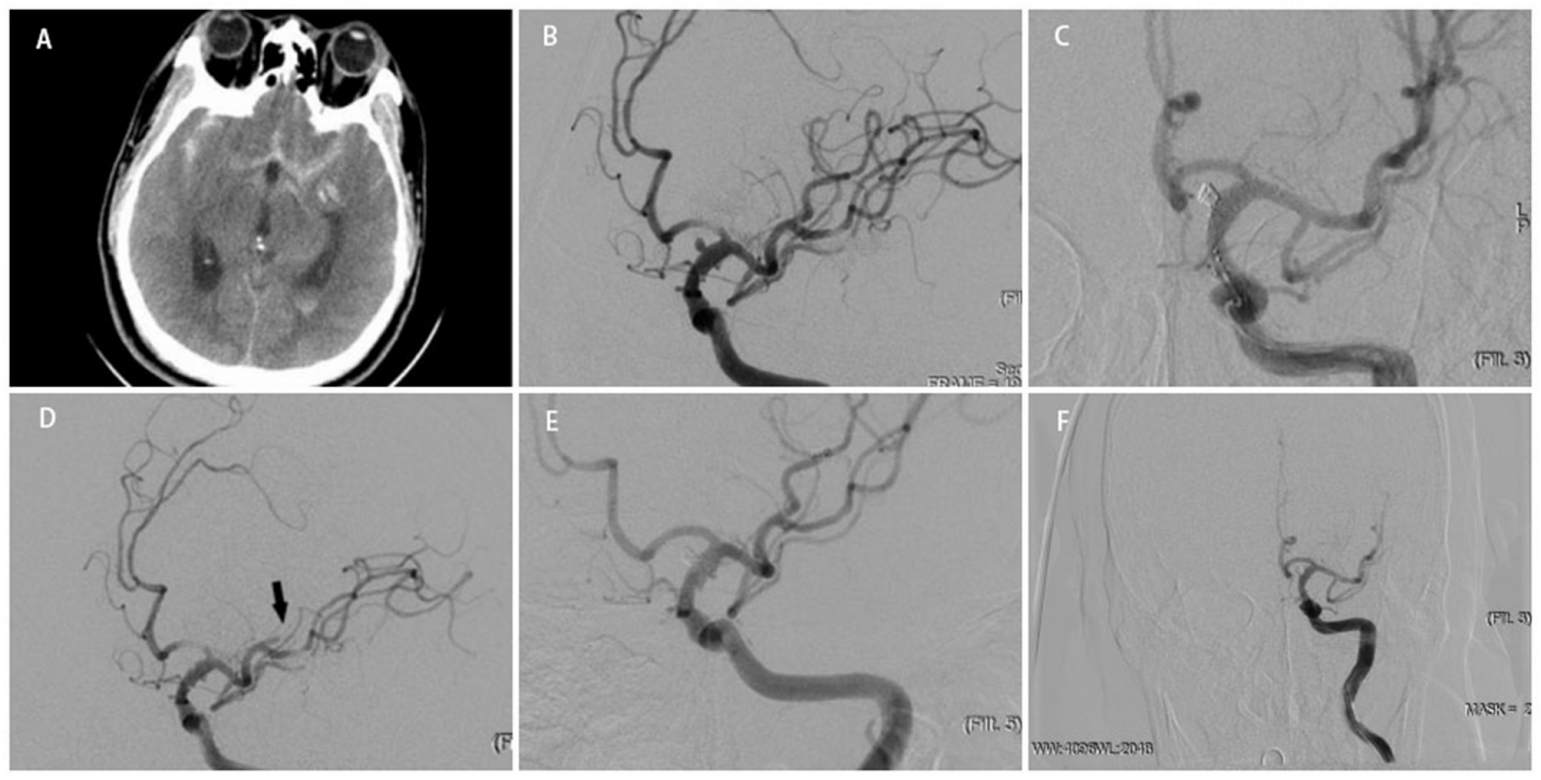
A middle-aged person was admitted to our hospital for sudden headache 10 h earlier (H-H grade 3). An emergency cranial CT revealed diffuse subarachnoid hemorrhage (A). Cerebral angiography at different angles showed a BBA located at the side wall of the C7 segment of right ICA. Multiple stents+coils got the nod to protecting anterior choroidal artery and posterior communicating artery from acute occlusion (B).Two LVIS stents (LVIS 4.5 ^*^ 20 mm) combined with coiling were delivered and deployed successfully, and the instant angiographic result revealed no contrast filling into the aneurysm (C). Intraoperative angiographic revealed that a branch of MCA occluded(black arrow) (D). After tirofiban injection, angiographic revealed the reappearance of MCA branch and total occlusion of the aneurysm (E). Angiographic follow-up at 5 months revealed total occlusion of the aneurysm (F).
